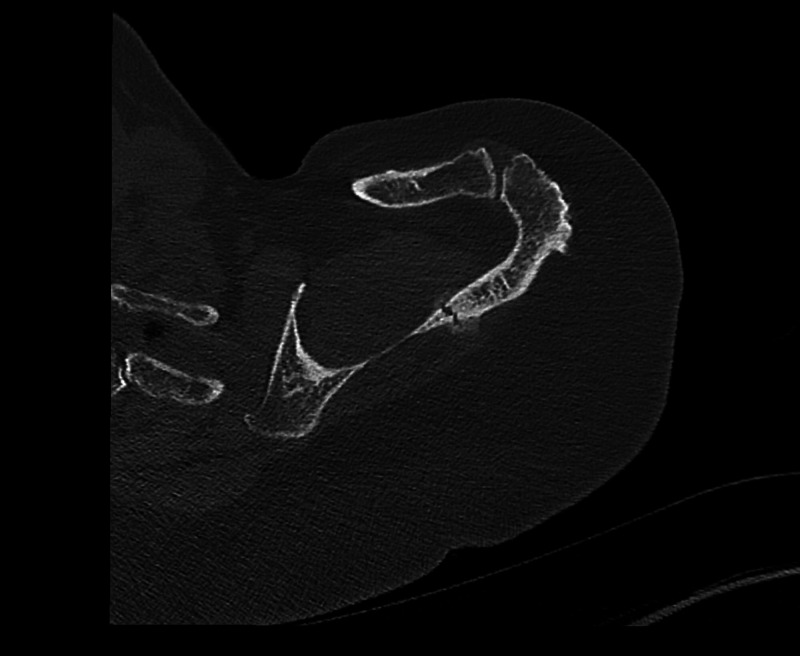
Axial section demonstrating a scapular spine fracture prior to EXOGENÒ therapy EXOGENÒ Ultrasound Bone Healing System, Bioventus, Durham NC, Netherlands
